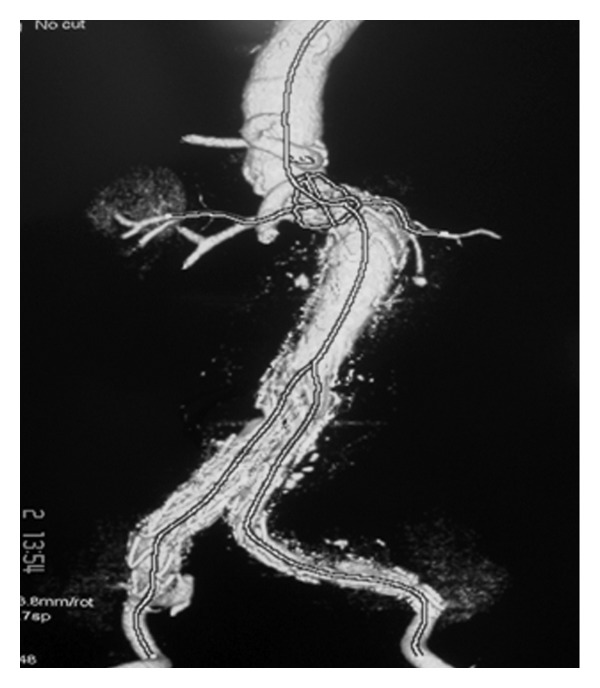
No endoleak was detected on CT angiography nine months postoperatively. The left limb of the graft is occluded, and the femorofemoral crossover bypass is patent.
